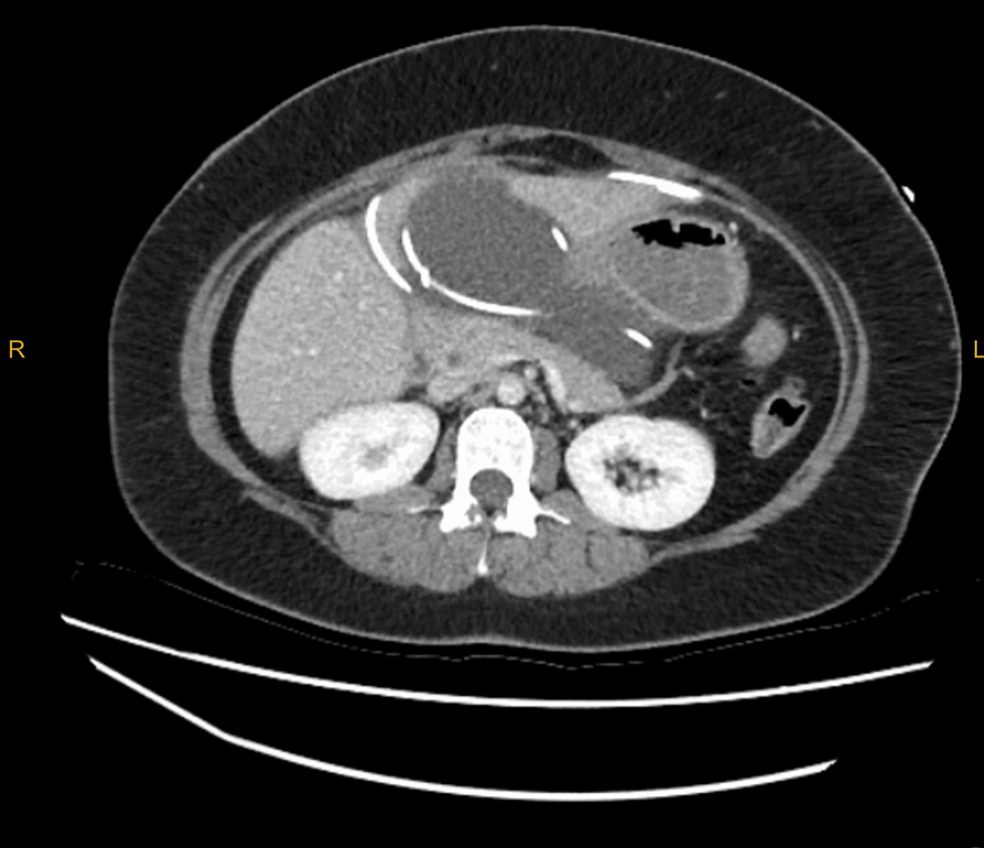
CT abdomen showing catheter tips within the collection
